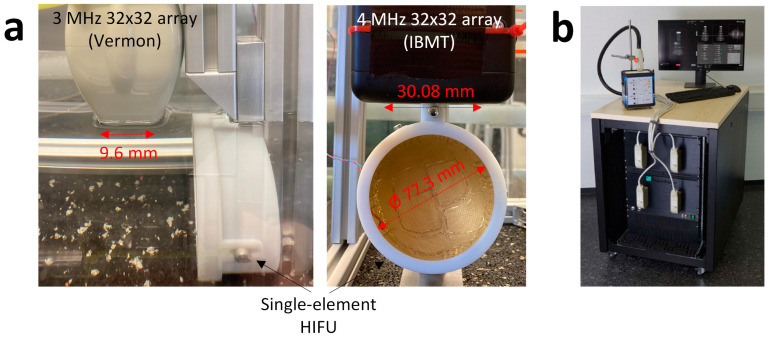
Experimental setup for the acquisition of cavitation signals in a water tank. (a) Side view of the 3 MHz 32 × 32 array and the single-element focusing transducer, as well as the front view of the 4 MHz 32 × 32 transducer and the single-element focusing transducer. (b) 1024 channel ultrasound research platform.
